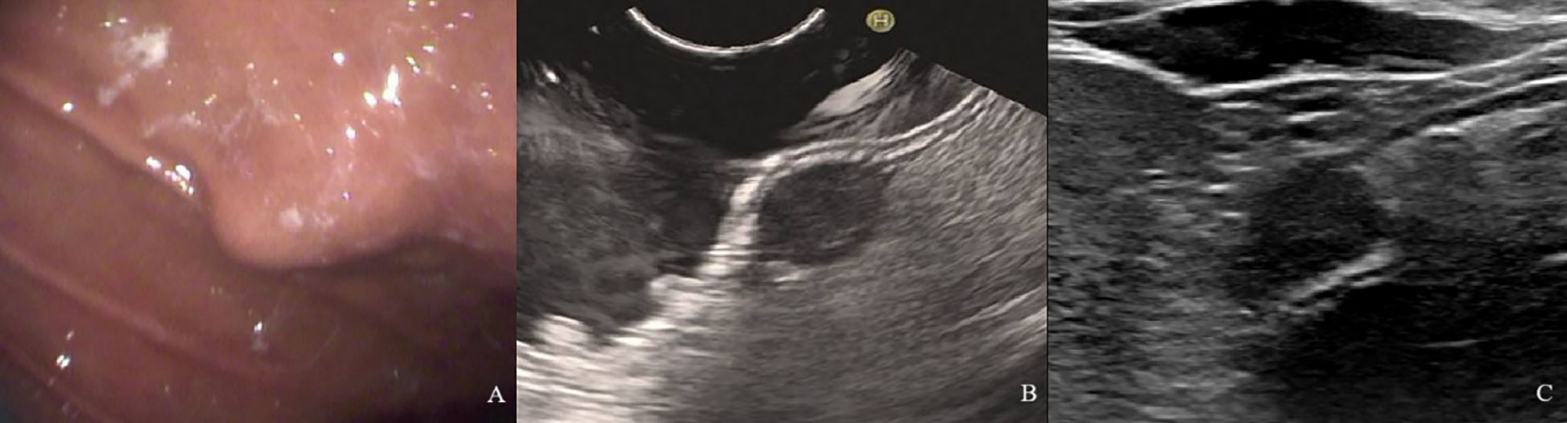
A 61-year-old woman with a 1.8×1.2cm GIST of low NIH risk stratification. (A) Gastroscopy showed a submucosal elevation with a smooth surface visible in the mid-lower anterior portion of the gastric body. (B) Endoscopic ultrasound showed a hypoechoic mass within the fourth layer of the gastric wall. (C) By oral contrast, ultrasound showed a hypoechoic mass in the fourth layer of the gastric body structure with clear borders and homogeneous internal echogenicity.
